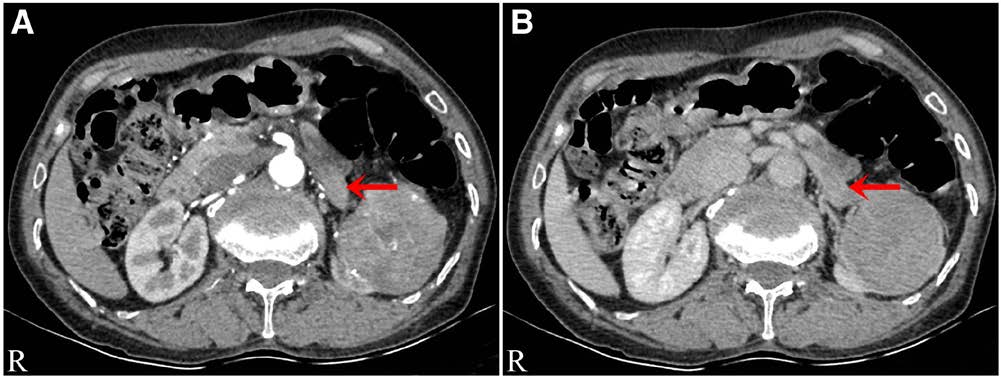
(A) The enhanced soft tissue shadow was found in the left renal vein in the arterial phase of the enhanced CT scan, as indicated by the arrow. (B) A filling defect was detected in the delayed CT scan, as indicated by arrows. CT = computed tomography
